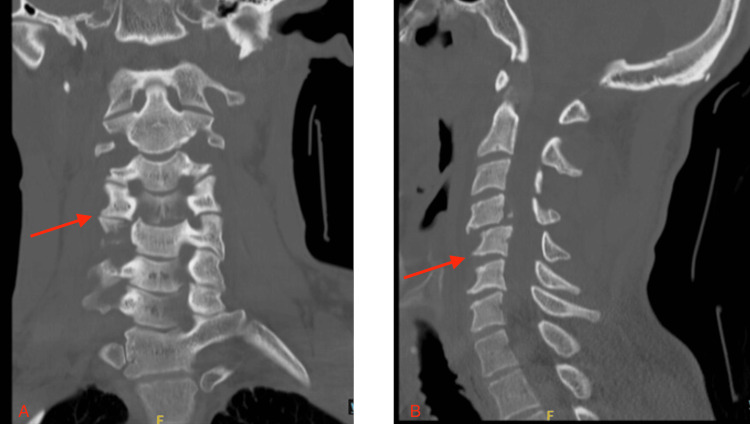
Cervical spine computed tomography (CT) anterior-posterior (A) and lateral (B) views. C4 inferior articular process with displacement of fracture fragment, C4 posterior inferior endplate with traumatic 5 mm anterolisthesis of C4 and C5. Trace anterior wedge deformity of the C5 vertebral body with acute fracture deformity.
